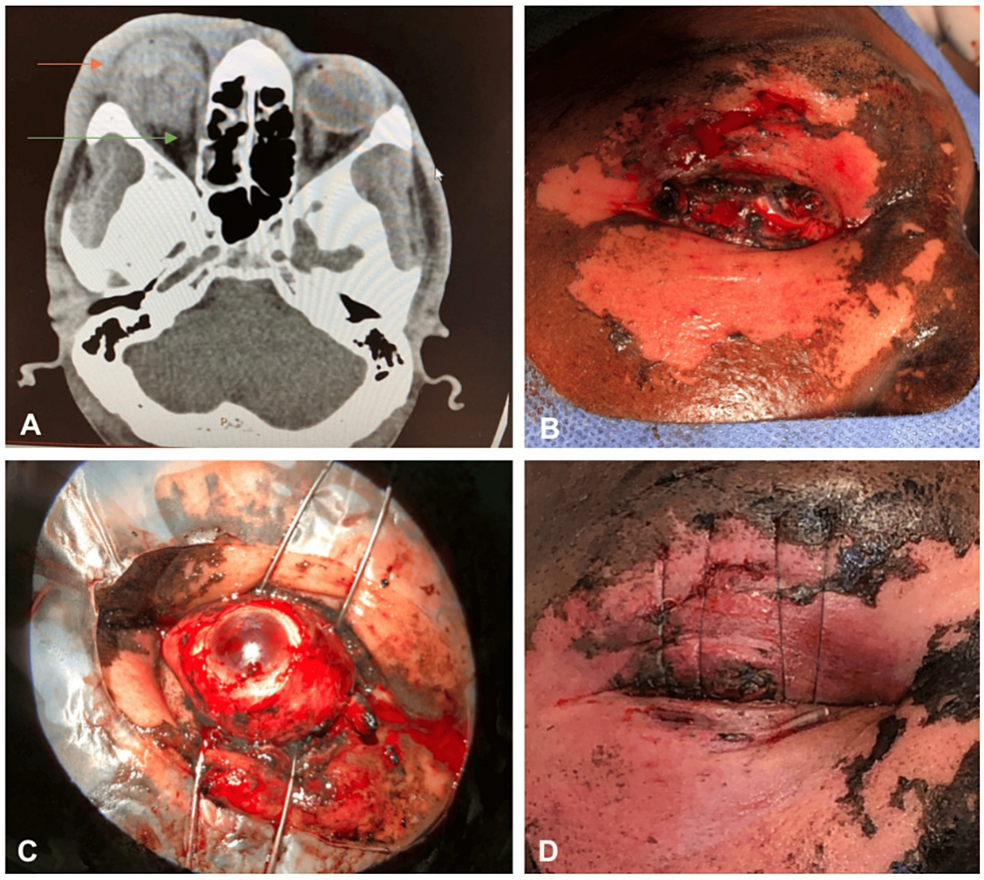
A 47-year-old male sustained an open globe injury after being struck by a firework (case 2). A: CT scan (sagittal view) showing rupture of the right globe posteriorly (green arrow) and vitreous hemorrhage (orange arrow). No intraorbital foreign body was identified. B: External photograph of the right eye. Right upper and lower lid burn injury and lacerations can be seen as well as second-degree burns to the right side of the face. C: Intraoperative external photograph of the right eye. There is diffuse subconjunctival hemorrhage, the cornea is hazy consistent with corneal burn injury, and hyphema is present. D: External photograph of the right lids following surgical repair and reconstruction. Modified Frost sutures were placed to achieve full corneal coverage and minimize cicatricial ectropion CT: computed tomography
